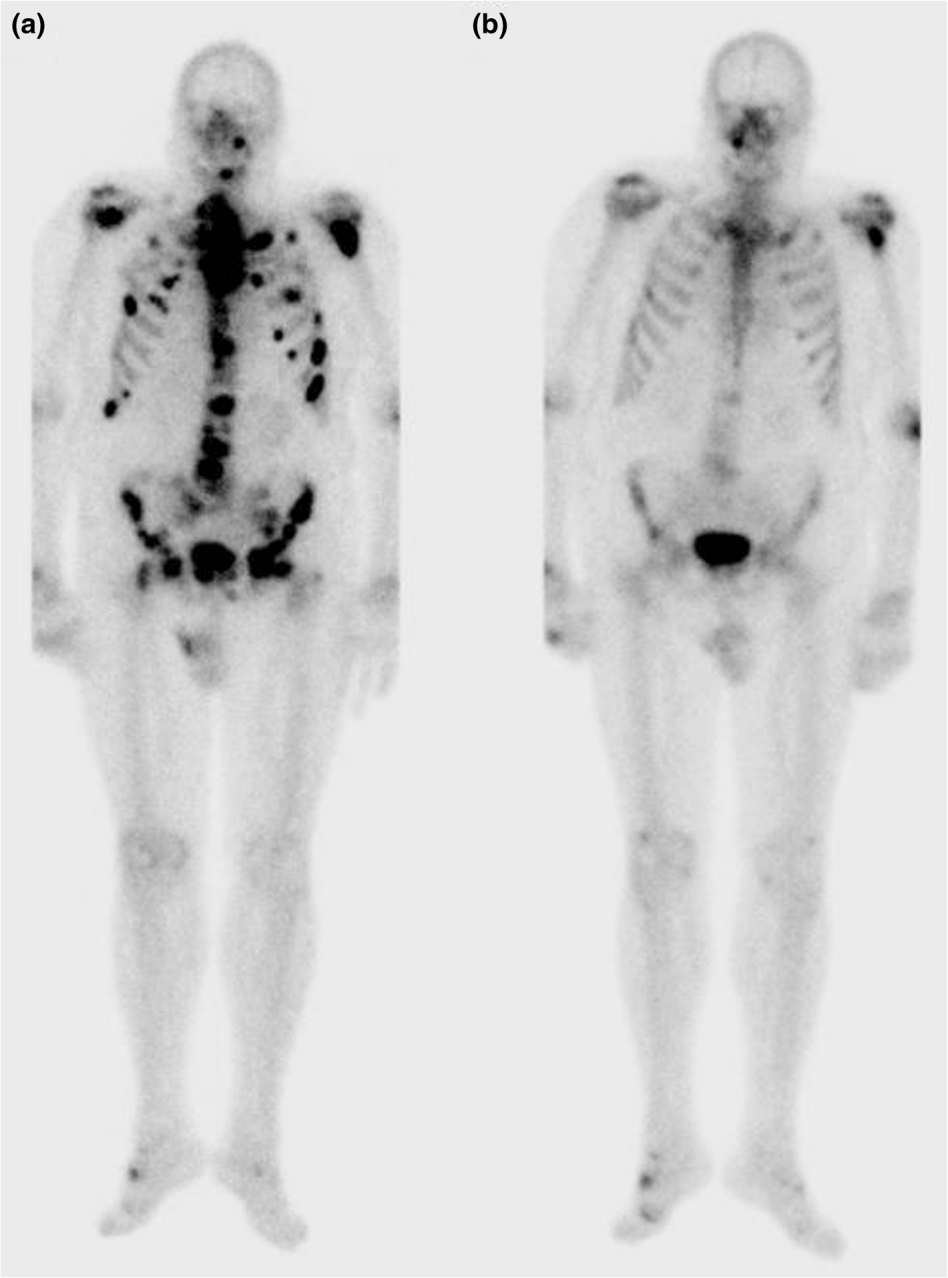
Pre- and post-androgen deprivation therapy images from planar bone scintigraphy (anterior view). Anterior images from planar bone scintigraphy of one patient at baseline (a) and after 6 months of satisfactory androgen therapy (b). PSA decreased from 92 ng/mL at baseline to 8.8 ng/mL (90 % reduction), and plasma-testosterone decreased from 1.7 to 0.07 ng/ml. All lesions initially suspected of malignancy in the axial skeleton demonstrated partial or complete regression, whereas lesions in large joints and small joints in the hands and feet were stable or progressed. The activity in the left elbow region is an artifact caused by contamination at tracer injection
